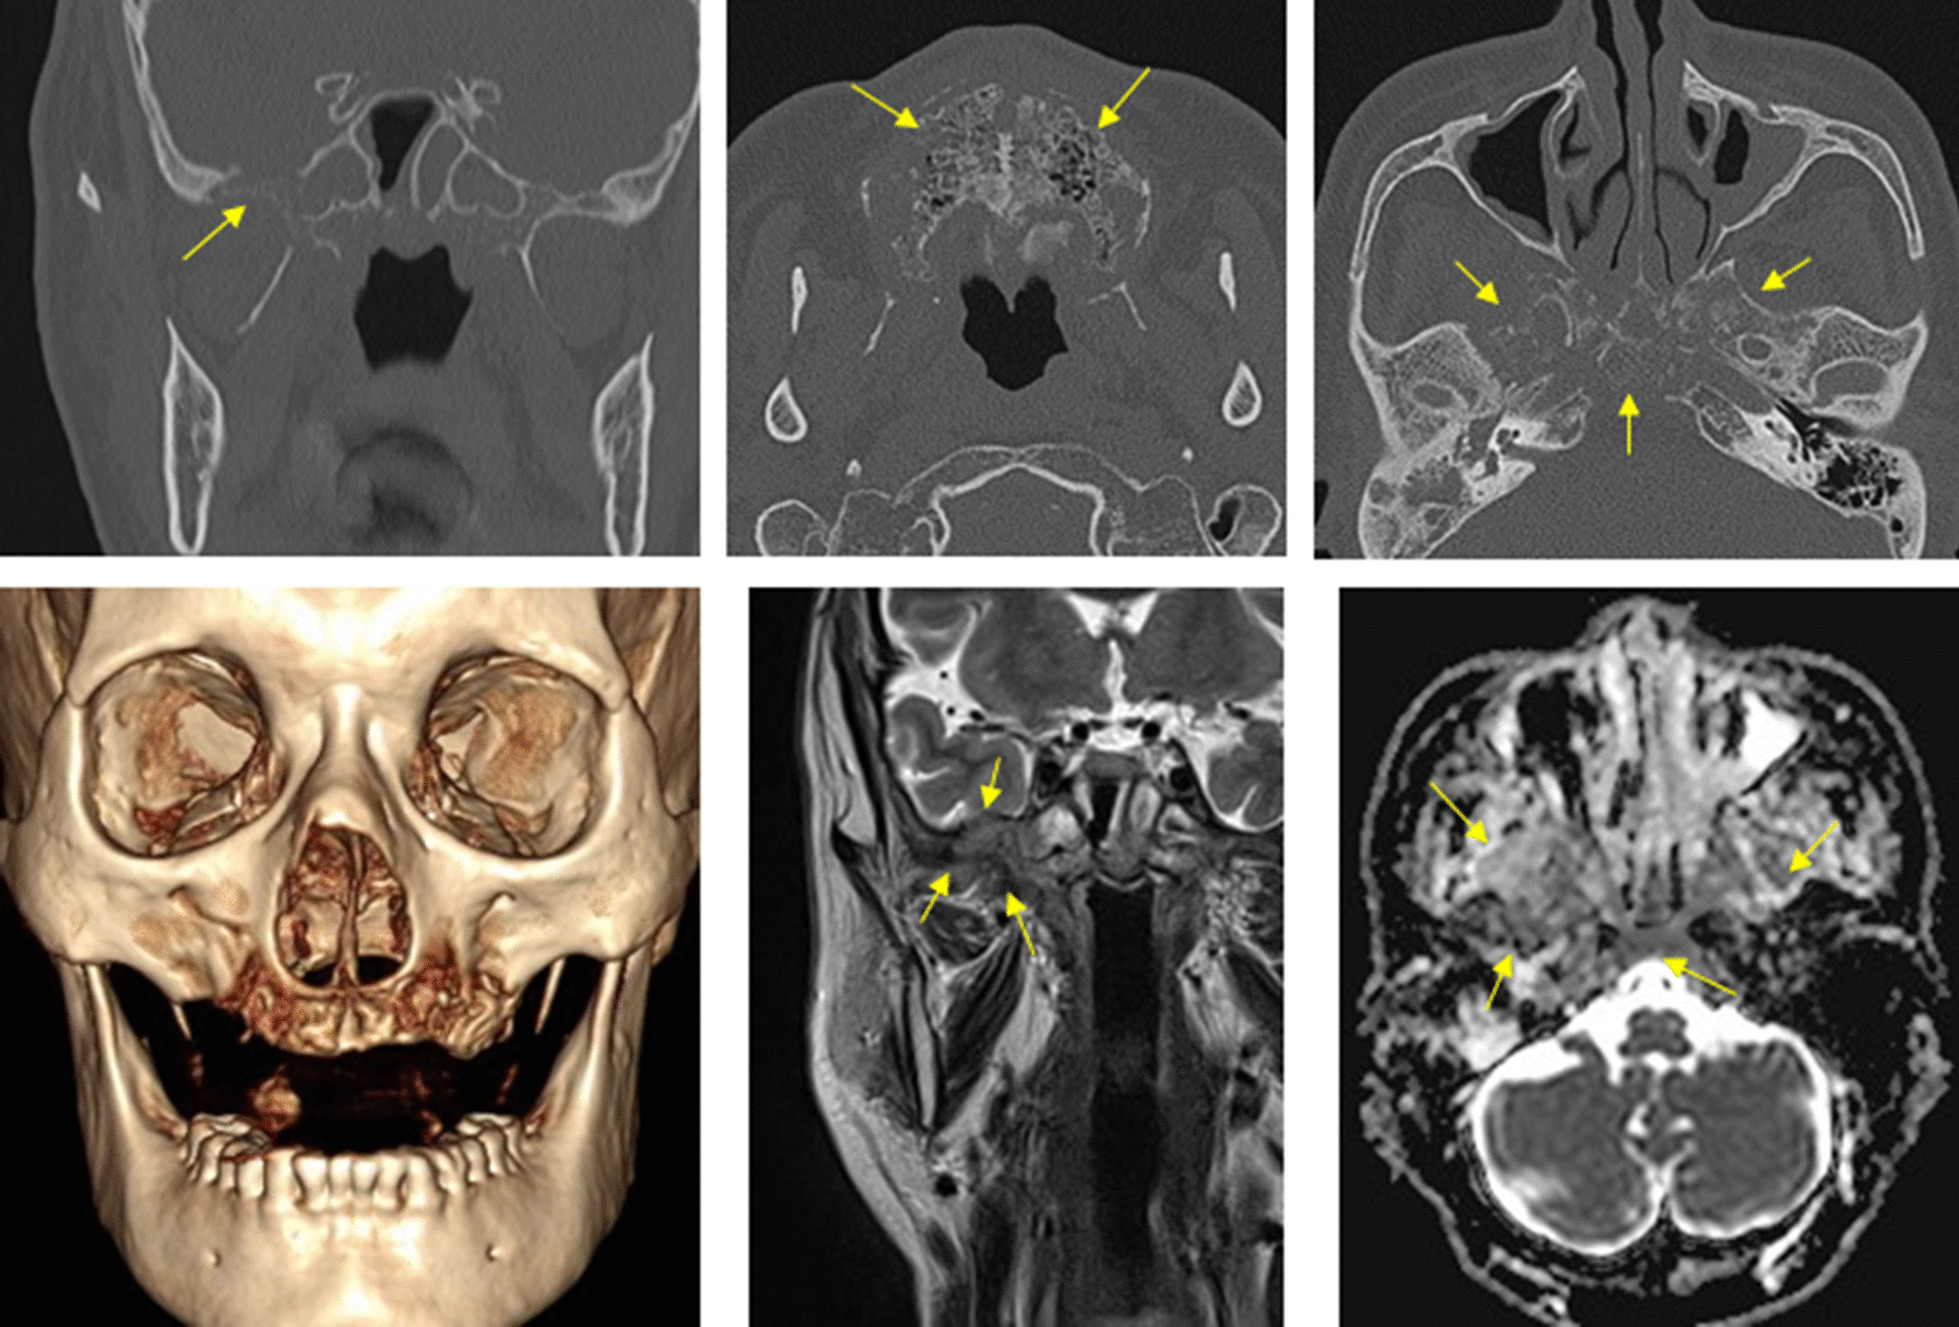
CT scan and MRI showing maxilla osteonecrosis. There is extensive osteolytic change with bone destruction of the central skull base including sphenoid body, sinus, right greater wing, and pterygoid process
